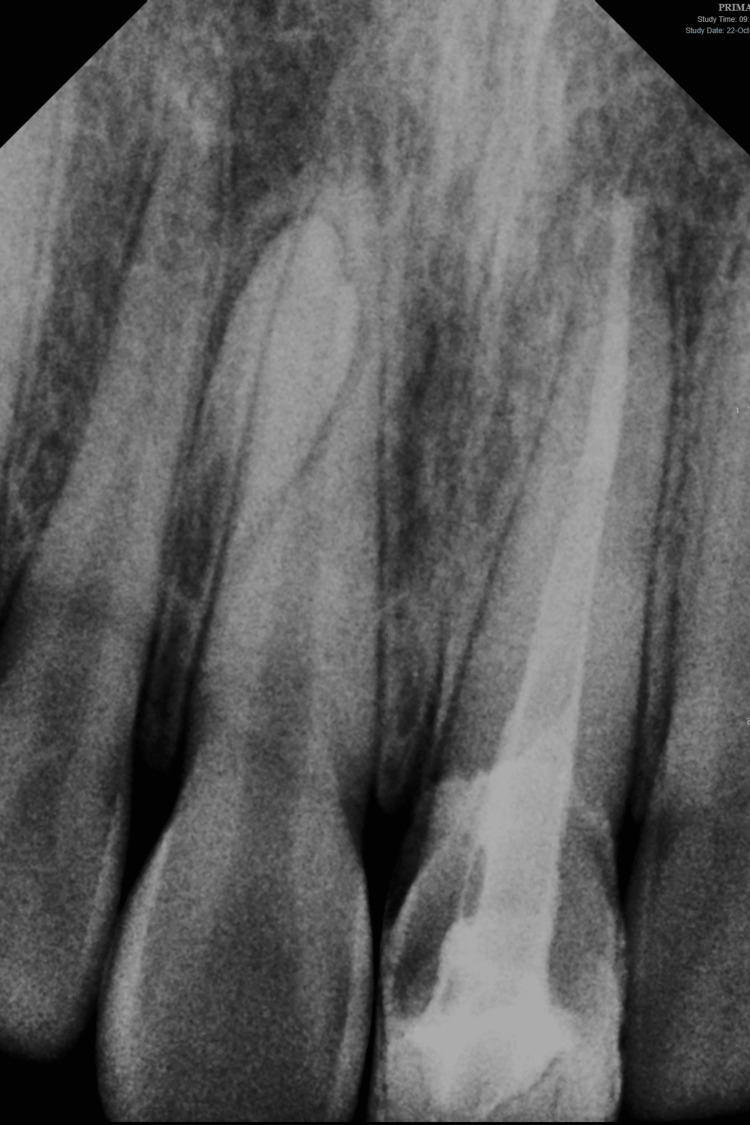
Periapical radiograph at one-month review
